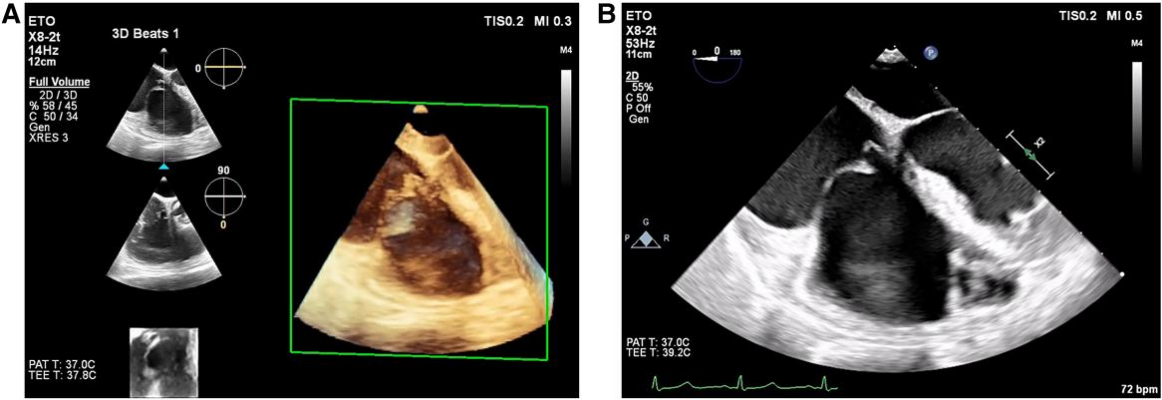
(*A*, *B*) Preoperative transoesophageal echocardiogram images.
